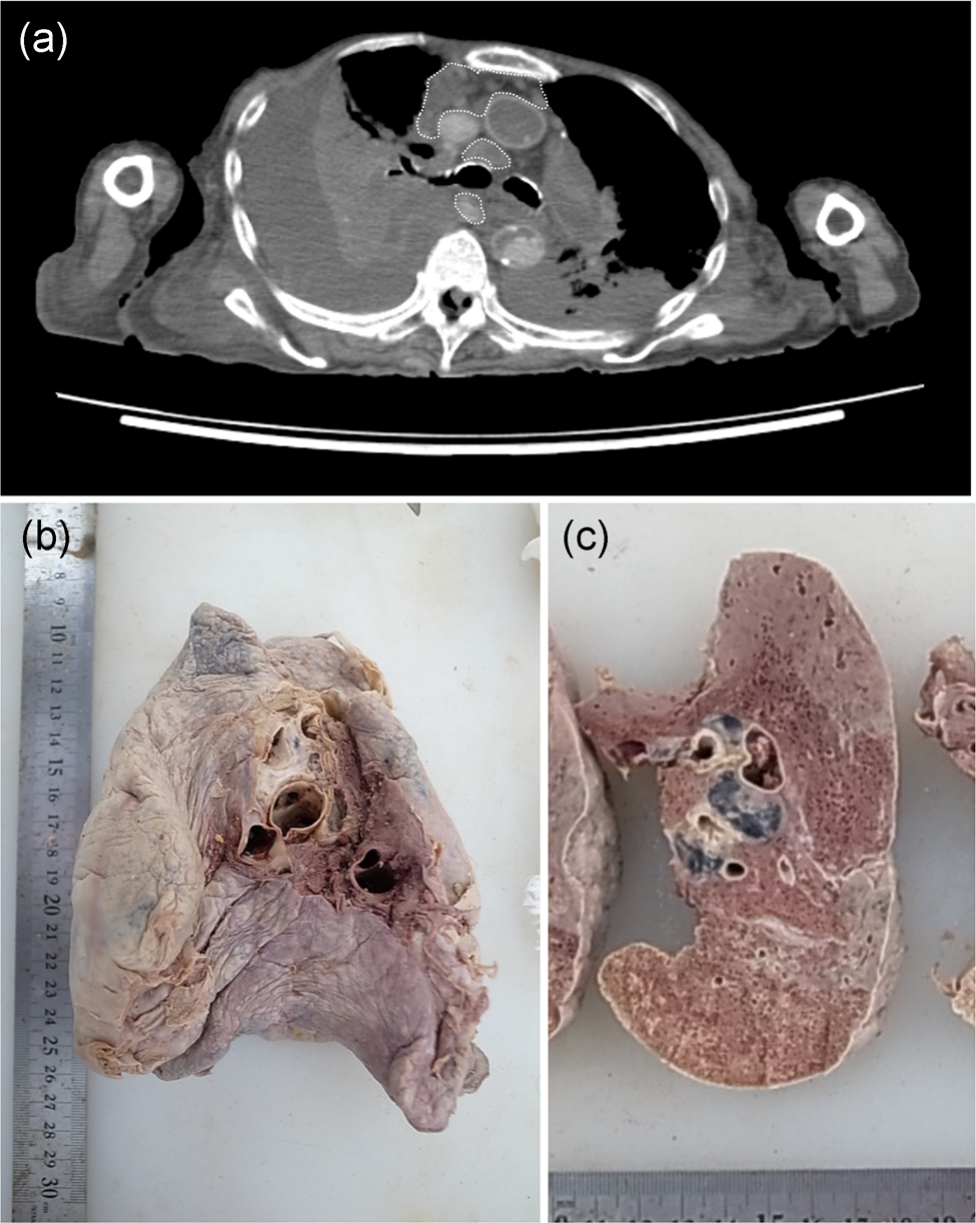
Postmortem imaging and gross appearance of anatomy Case No. 20. Chest PMI‐CT shows that mediastinal lymph nodes and right hilar lymph nodes are markedly swollen (dotted line area) (a). A pleural effusion with right‐sided predominance is also seen. The macroscopic view of the right lung (b) and the cut surface of the right lung at the hilar level (c). A swollen hilar lymph node is seen. PMI‐CT, postmortem imaging‐computed tomography.
